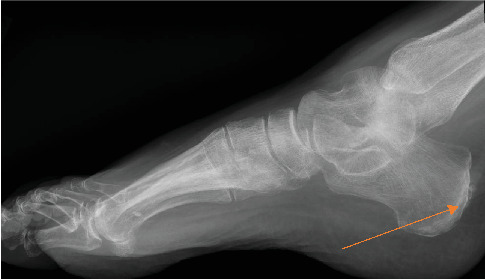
X-ray of the left foot demonstrating degenerative changes and mild retrocalcaneal spur (orange arrow).
